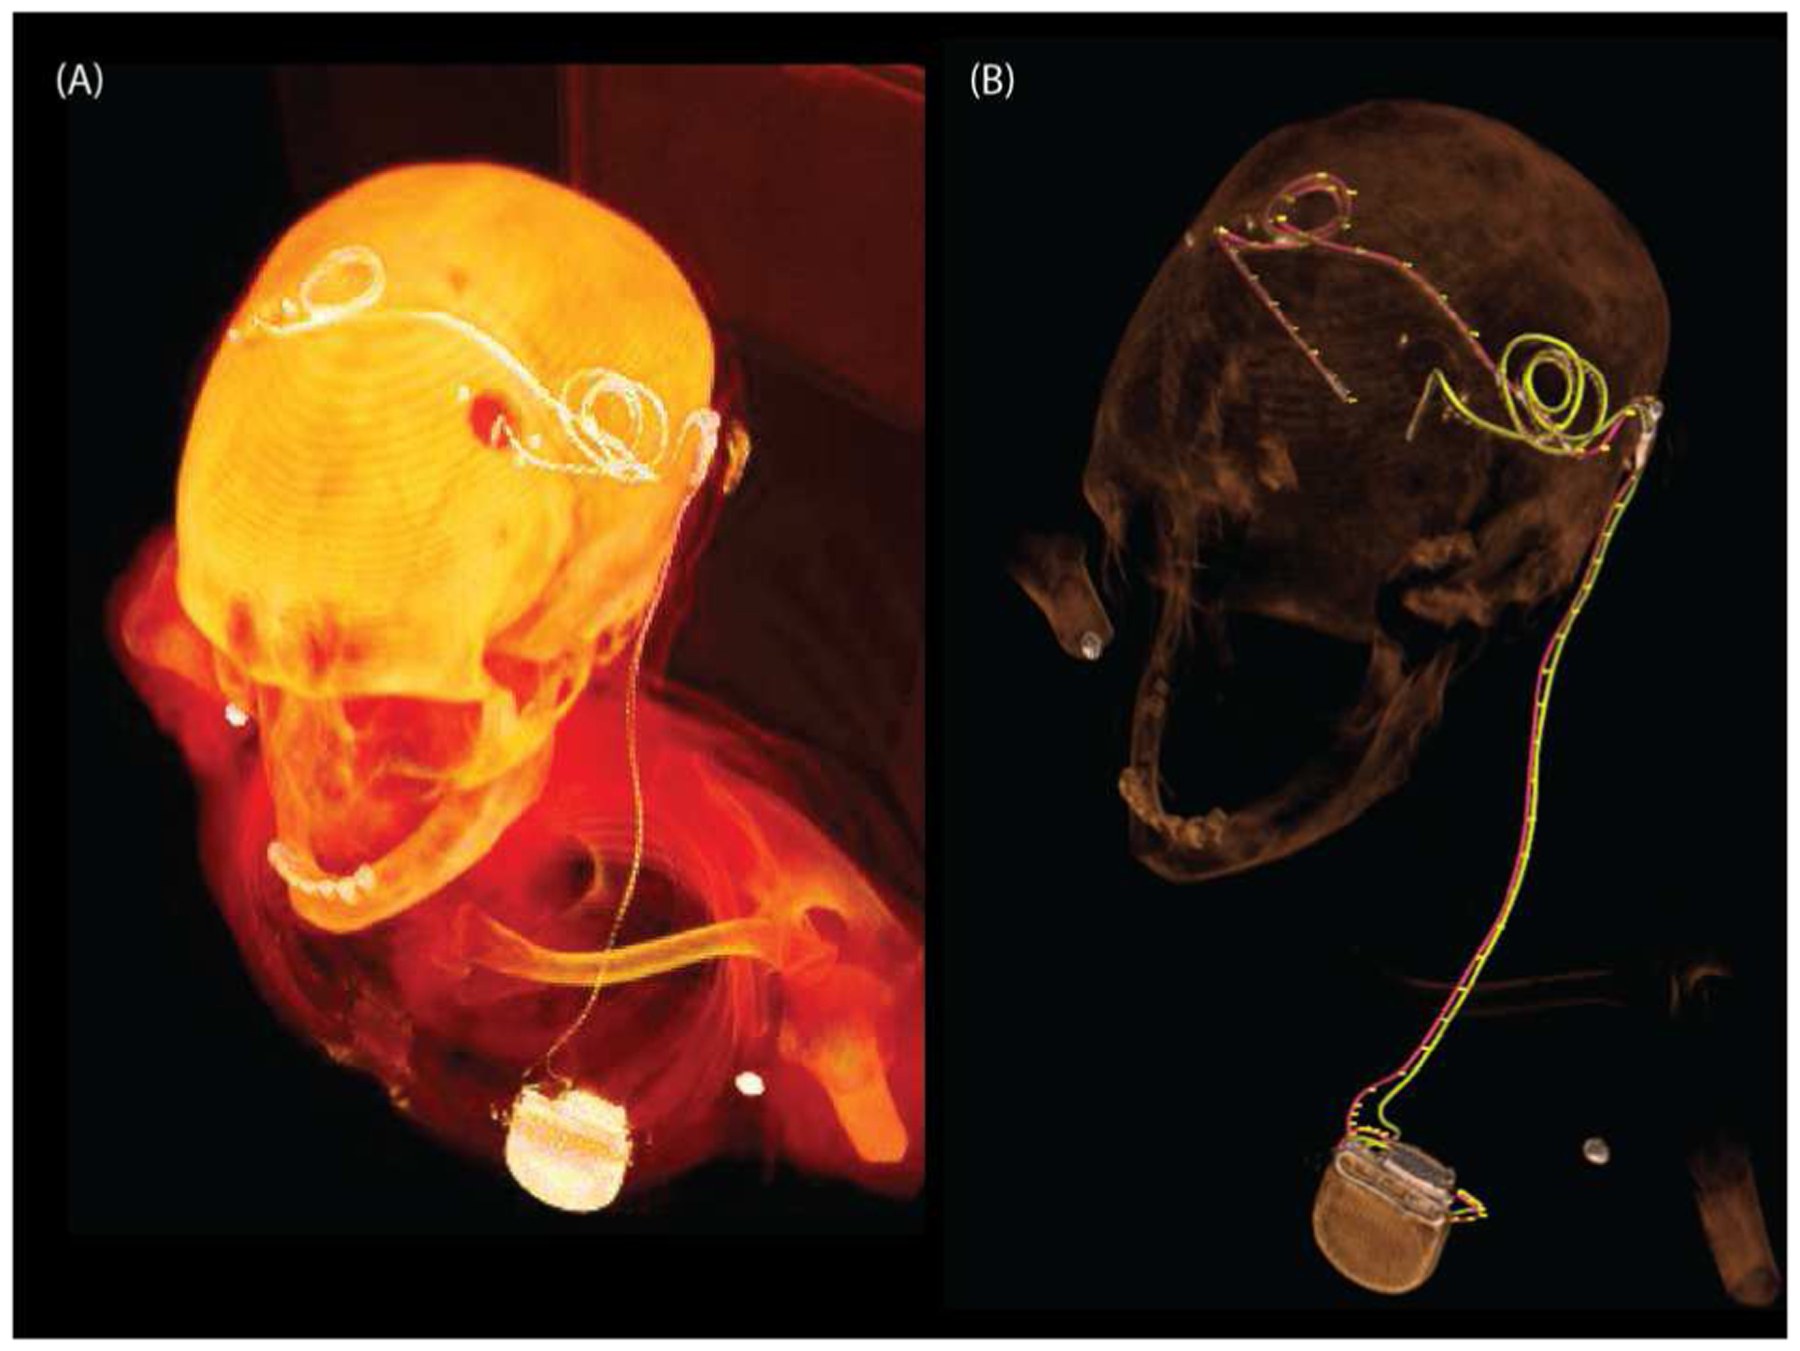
3D rendered view of CT image of a DBS patient with two DBS leads connected to single IPG (left) and segmented trajectories over the rendered view (right).
